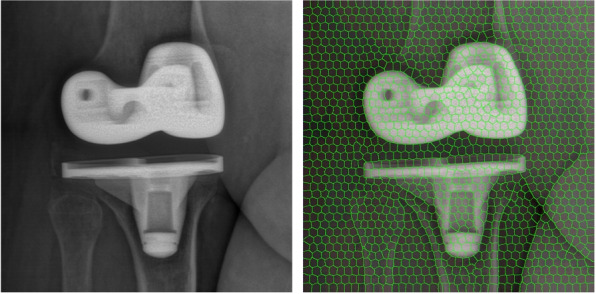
Example of label-based pre-processing step : input X-ray image (left) and pre-processed image (right)
